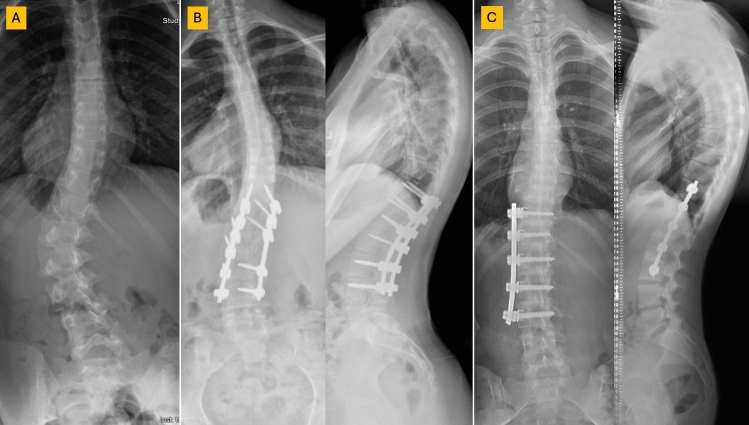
Radiographic examples of adolescent idiopathic thoracolumbar scoliosis (A; Lenke Type V) treated with anterior instrumented fusion (B) or posterior instrumented fusion (C)
